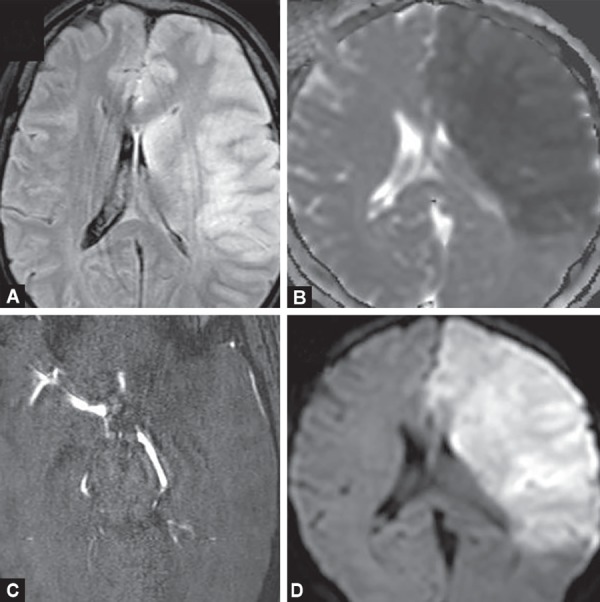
Large acute left MCA territory infarct appearing hyperintense on FLAIR (A) and showing diffusion restriction; (B) Loss of flow related enhancement in the left MCA in the TOF angiogram; (C) suggests occluding thrombosis (D) with low ADC values on ADC map
